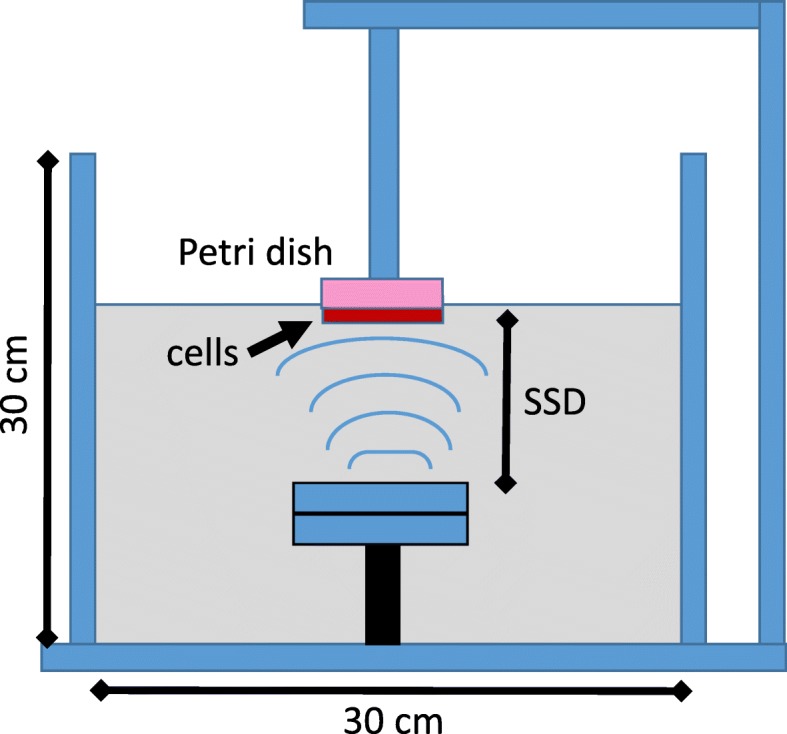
Ultrasounds setup. For VLIUS exposure, a line of ultrasonic signal was generated by a piezoelectric unfocussed transducer immersed at the bottom of a tank filled with degassed water, powered to a signal generator (Agilent 33220A) and a signal amplifier (Amplifier Research 25A250). A sinusoidal signal at the frequency of 1 MHz was generated and measured by a needle hydrophone (S.N. 2090, Precision Acoustics) of 0.5 mm diameter with a sensitivity of 483 mV/MPa at 1 MHz, connected to an oscilloscope (Tektronix TDS 3052B). Continuous ultrasound exposures in terms of ‘spatial peak temporal average intensity’ (Ispta) equal to 40, 80, 120 and 150 mW/cm^2^ were administered for 15 min on a petri dish (60 mm), submerged up to half of its thickness and aligned coaxially with the transducer, at a fixed distance (Source-dish Surface Distance (SSD) from the transducer
